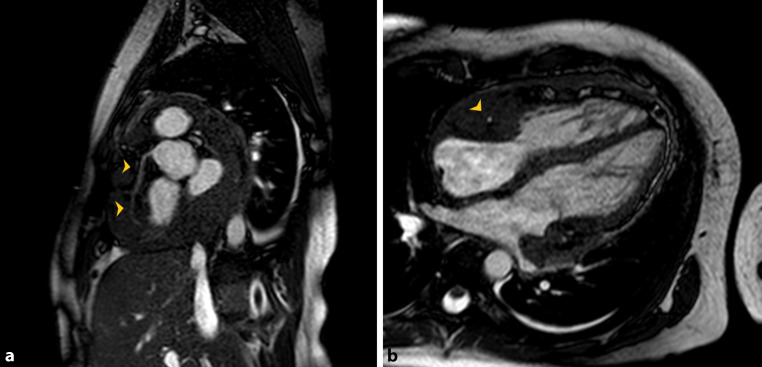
Cardiac magnetic resonance imaging—short axis view (a) and 4‑chamber view (b) showing the course of the right coronary artery inside the tumour mass (*arrow*)
